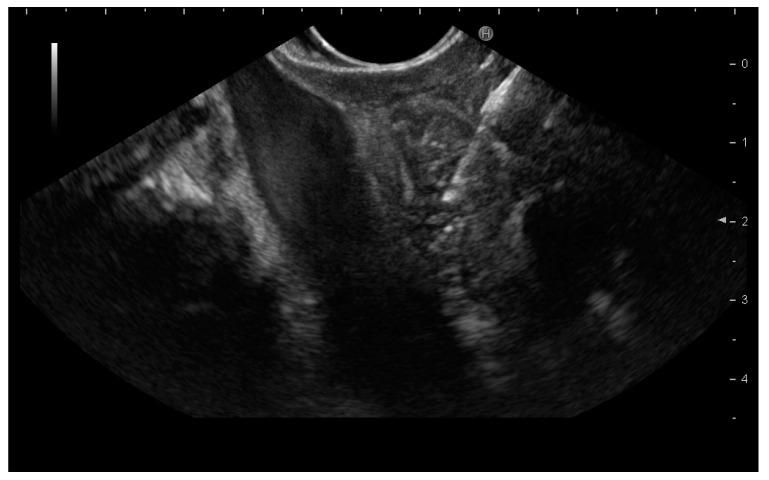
Endoscopic ultrasound-guided lymph node sampling.
